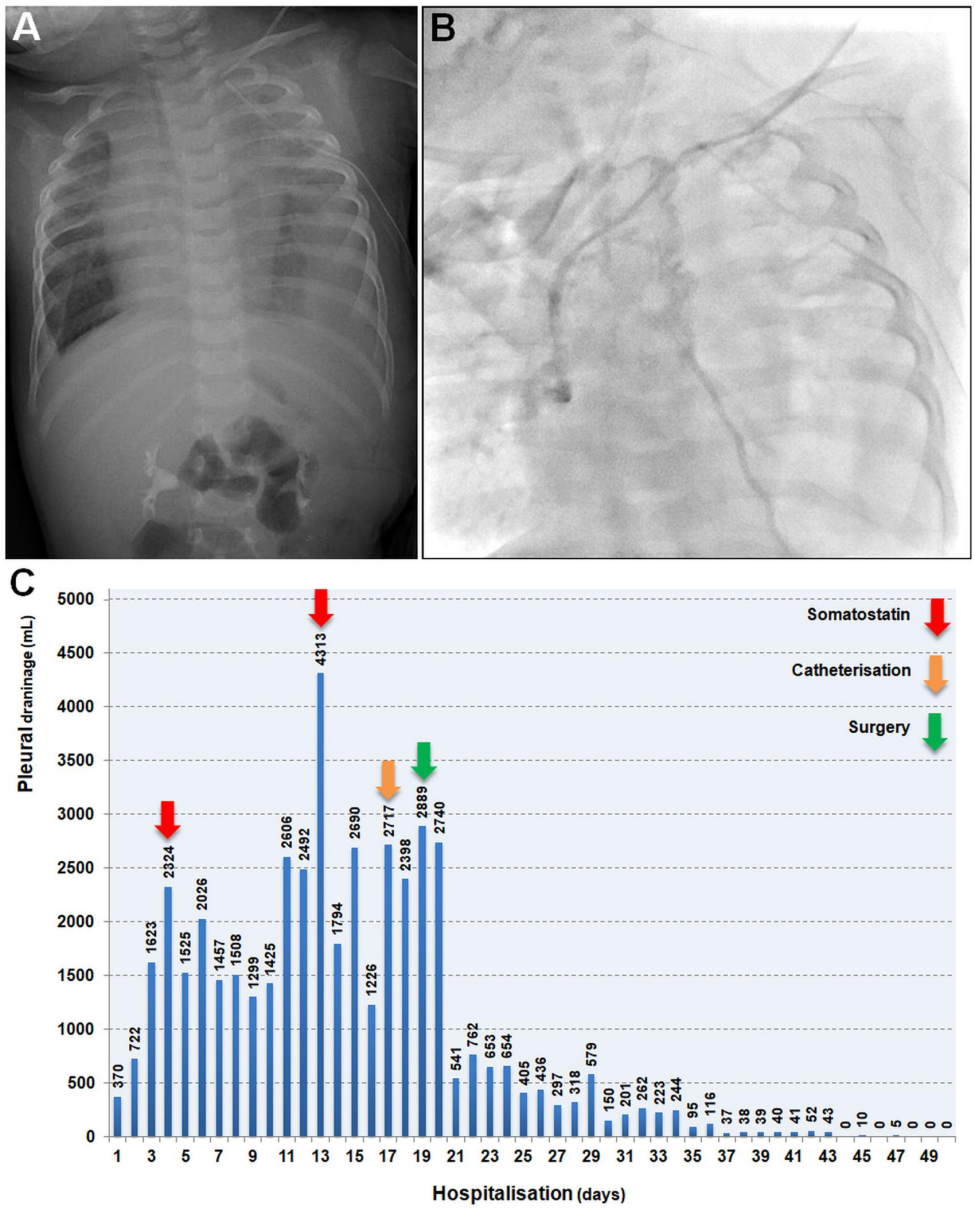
Preoperative chest X-ray revealing left massive chylothorax (A) and catheterization showing occluded left Pirogoff angle and innominate vein (B). (C) The amount of chylous pleural drainage along with initiation points of treatment modalities on the last admission.
